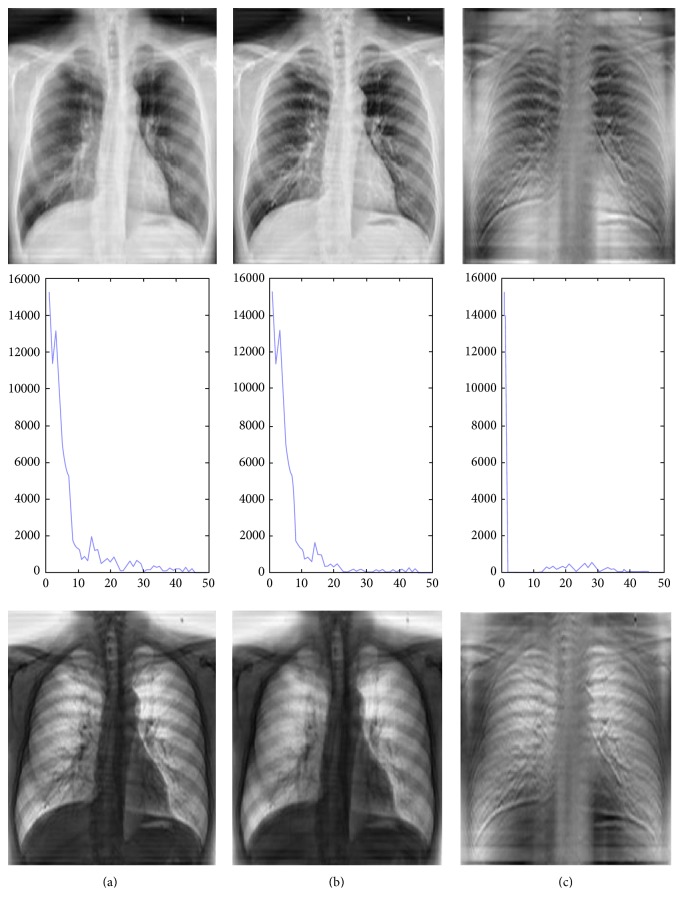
(a) From top to bottom: the chest X-ray image, the image frequency curve, and the chest X-ray image with inversed gray scale. (b) From top to bottom: chest X-ray image by Butterworth filtering, the image frequency curve, and the chest X-ray image with inversed gray scale. (c) From top to bottom: lung texture image reconstructed by the filtered frequency information, the frequency curve, and the lung texture image with inversed gray scale.
